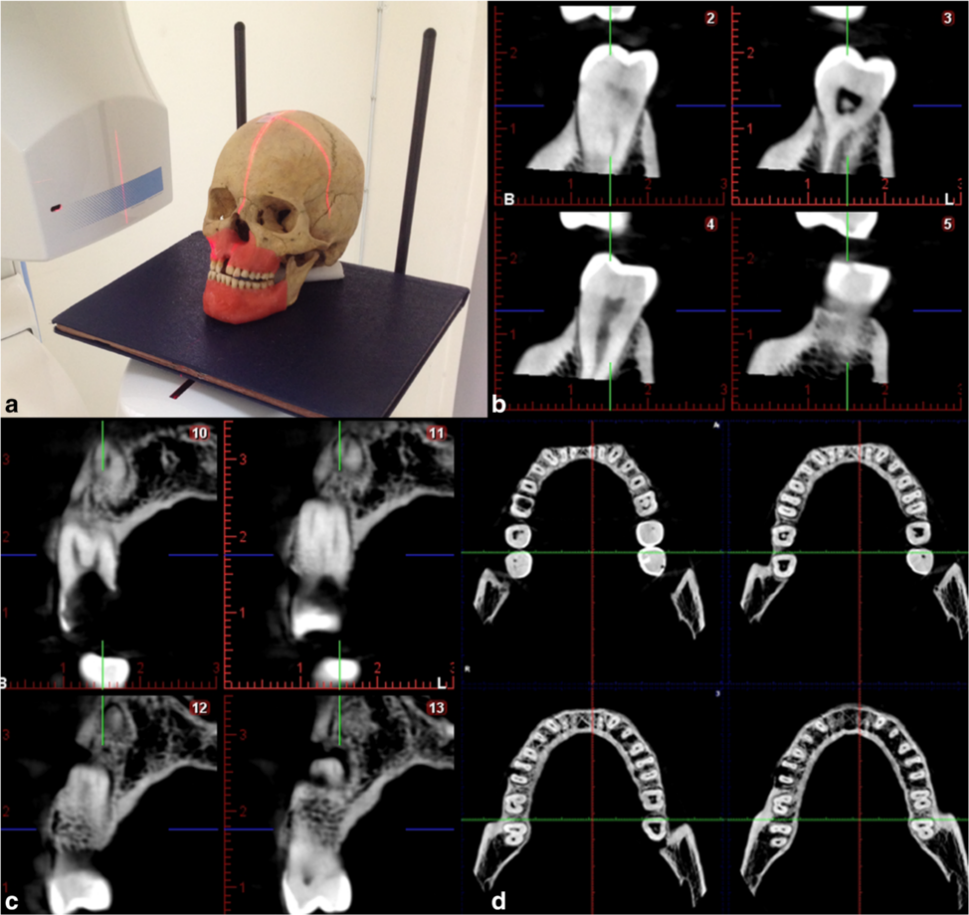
CBCT images showing (a) the position of the skull in the machine, (b,c) the periodontal defects in cross sections, (d) and axial planes
